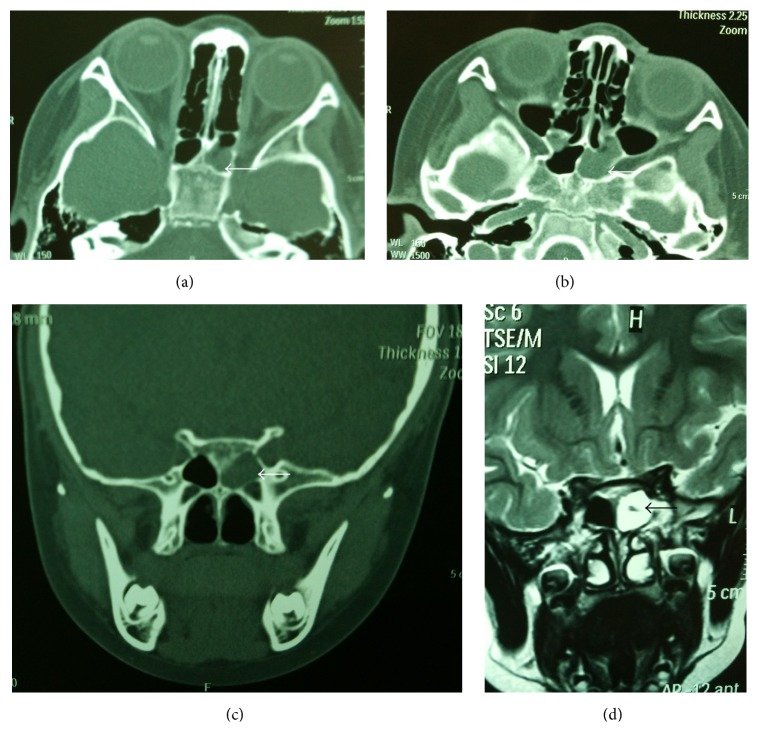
(a and b) Axial CT scans showing only sphenoid sinus with soft tissue density shadows on the left (*white arrow*). (c) Coronal CT scans of a patient with soft tissue density shadows in the left sphenoid sinus (*white arrow*). (d) T2-weighted MRI shows hyperintensity in the left sphenoid sinus (*black arrow*).
